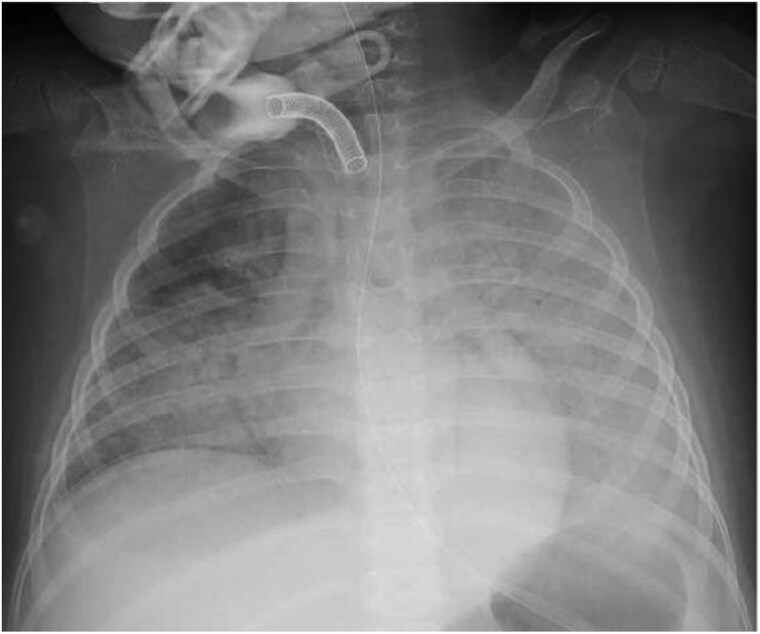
Chest radiograph taken during the onset of transient heart failure. Severe pulmonary congestion suggestive of heart failure was observed.
